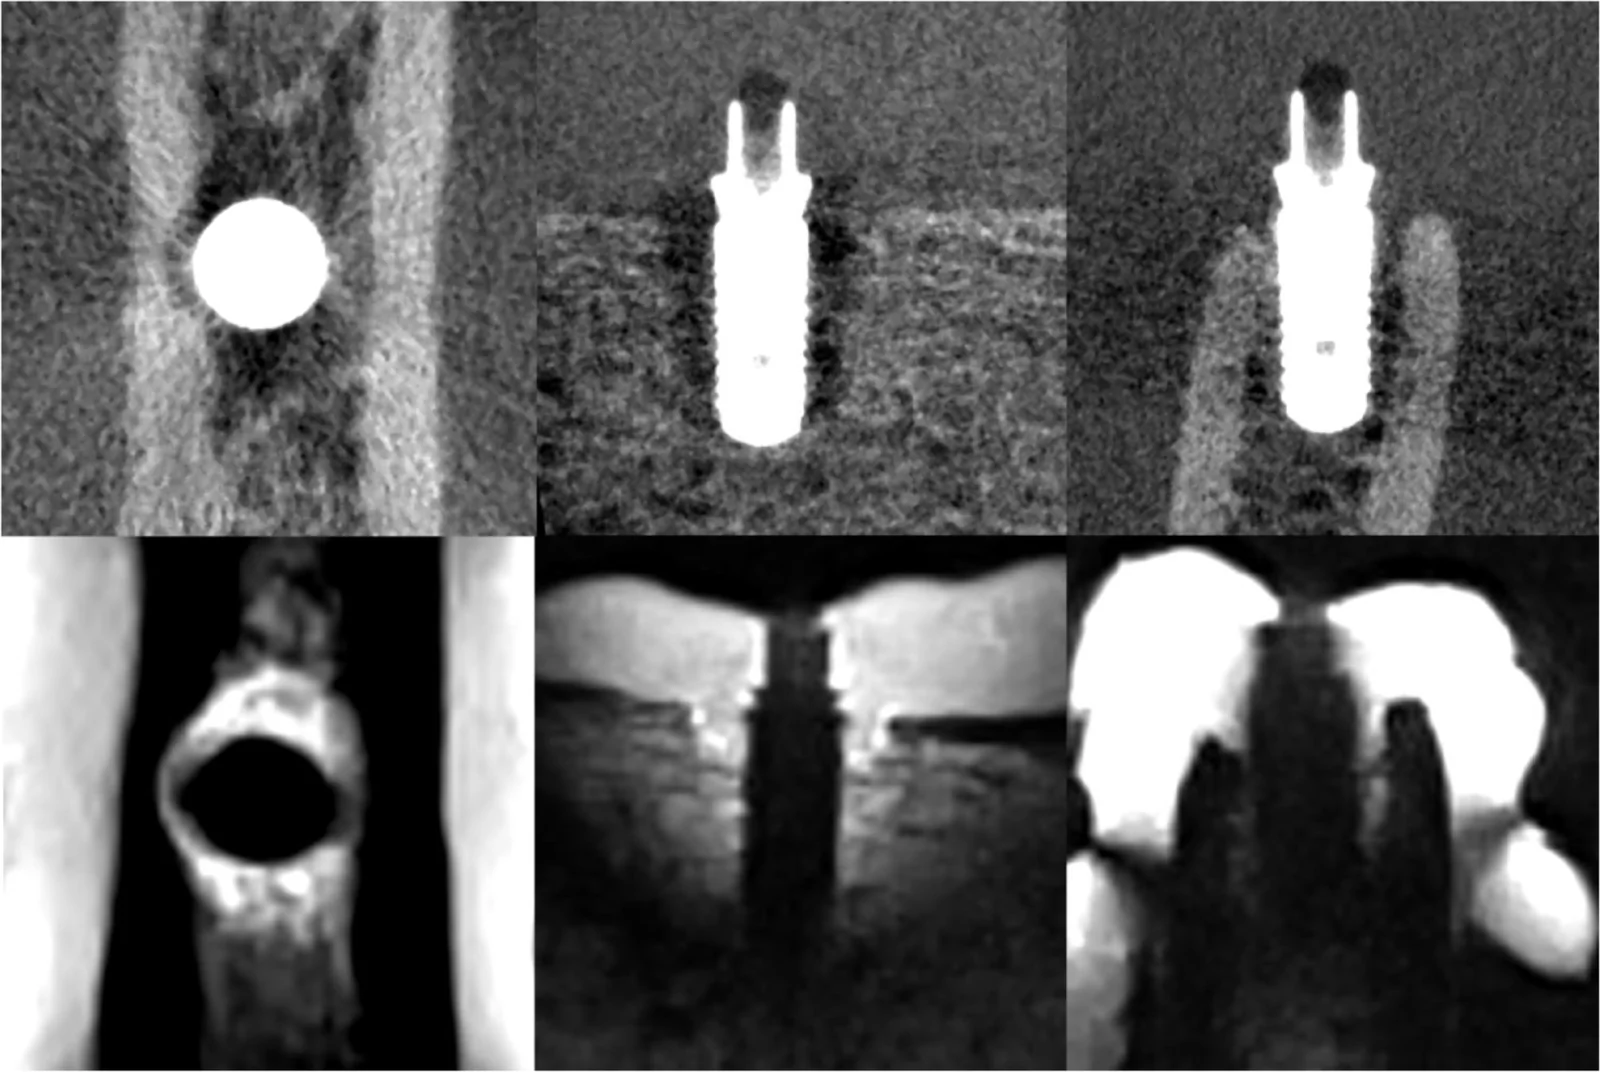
Examples of large 4-wall defects in CBCT/dMRI (upper/lower row) in axial, sagittal, and coronal reconstruction (left to right)
